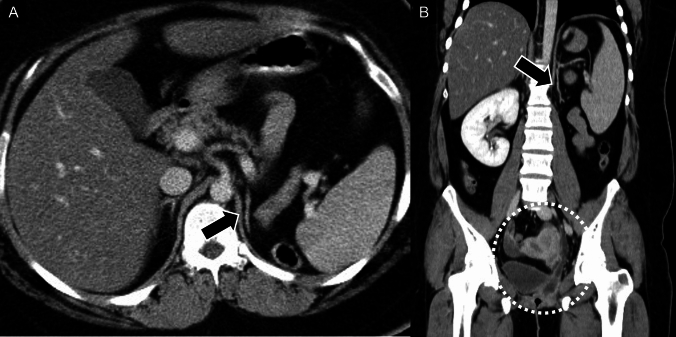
Discoid adrenal gland in a patient with Herlyn–Werner–Wunderlich syndrome. Computed tomography imaging in a 20-year-old female presenting with amenorrhea showing a bicornuate uterus with a cystic structure continuous, with the cervix on the left side of the pelvic floor (B: white dotted circle), along with agenesis of the left kidney. The left-adrenal gland is flattened and elongated, displaying the characteristic discoid shape (A, B: black arrow), which is commonly associated with unilateral renal agenesis
